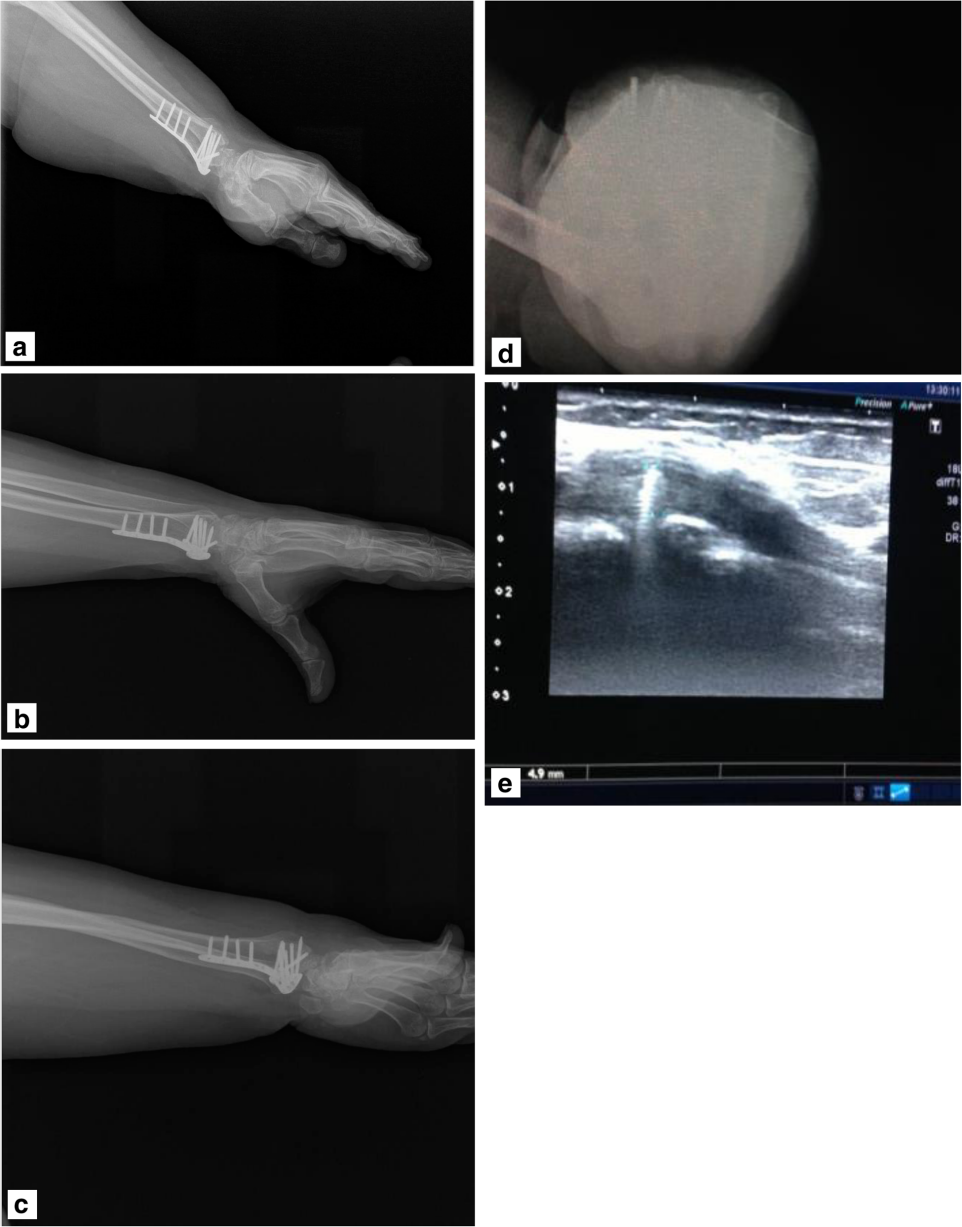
Patient number 8. Left wrist fracture, second compartment, 4.9 mm penetration. Postoperative lateral, 45° supination, 45° pronation, dorsal tangential and USG images. a Lateral. b Penetrating screw was not detectable on the 45° pronation radiograph. c Penetration was barely seen on the 45° supination radiograph. d Screw penetration on tangential radiograph. e 4.9 mm penetrating screw in the second compartment was seen by ultrasonography
